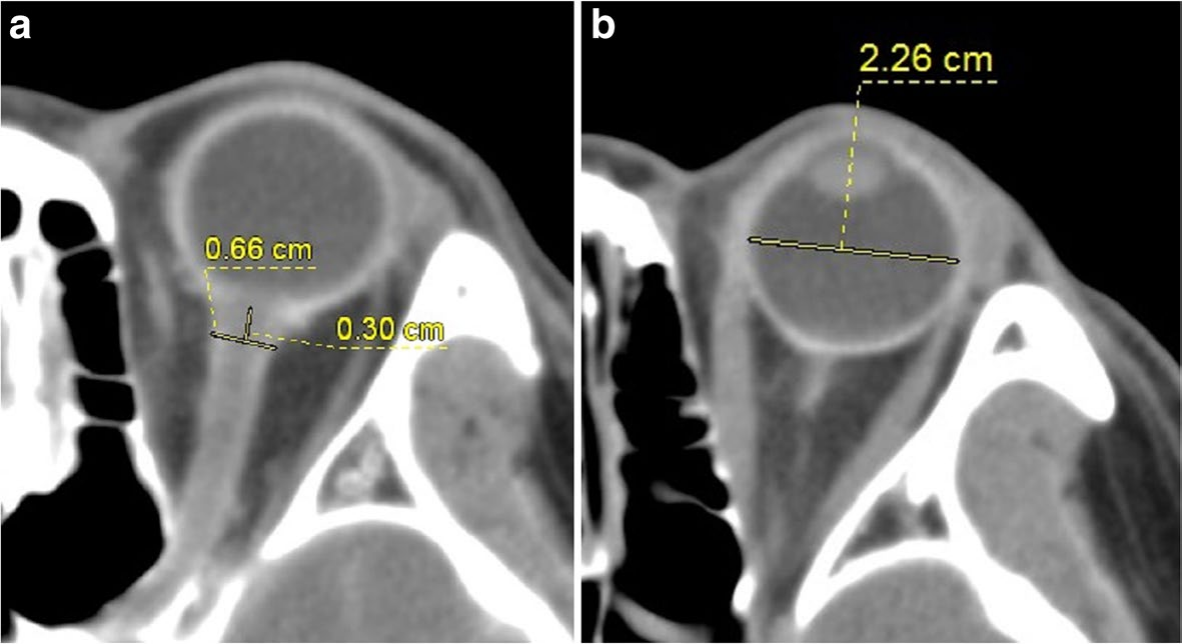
A Optic nerve sheath diameter (ONSD) measurement by head CT scan. B Eyeball transverse diameter (ETD) measurement by head CT scan
